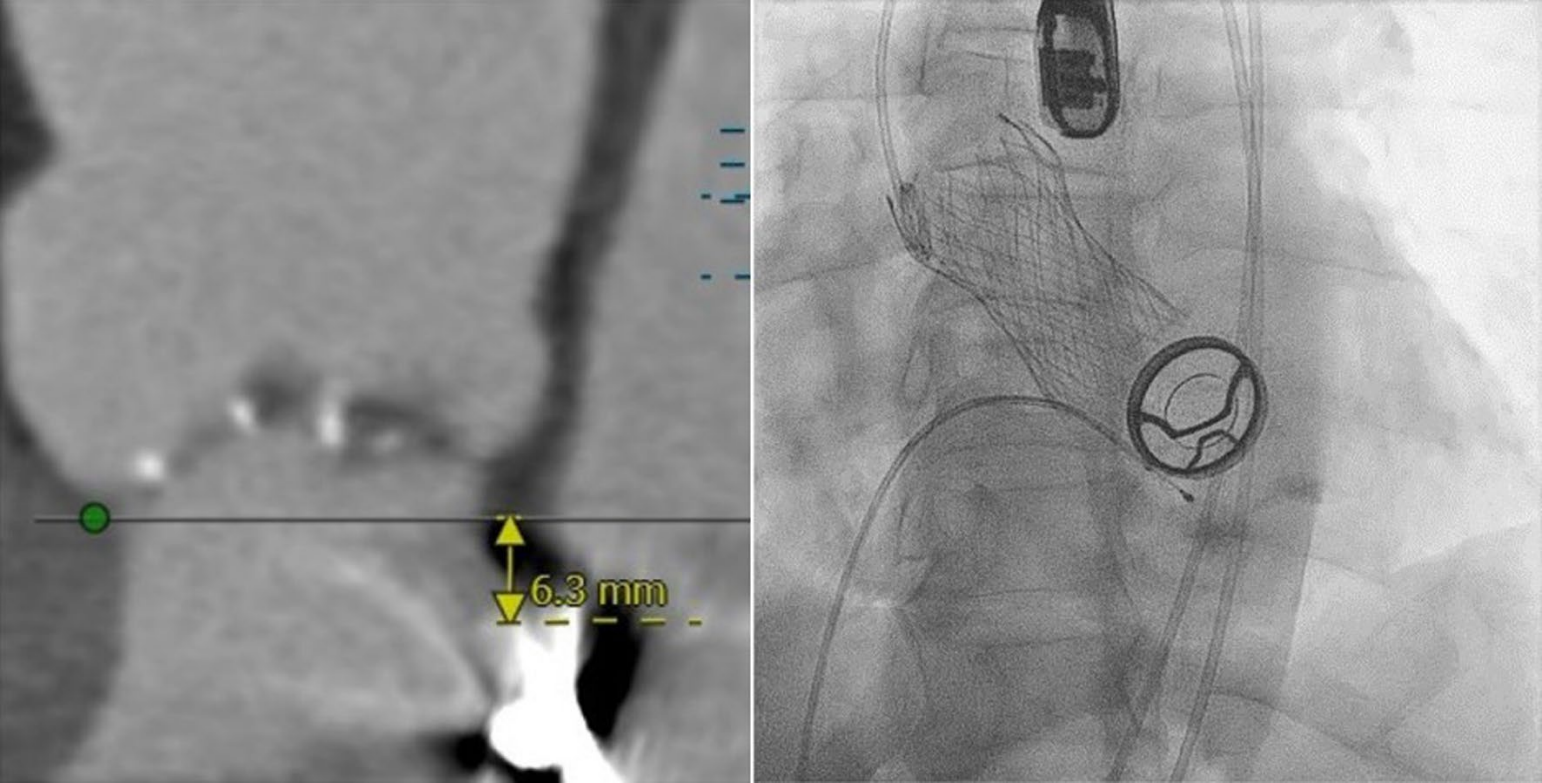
(case 1): On the left, pre-procedure Cardiac CT scan showing the prosthetic mitral valve—aortic annular distance. On the right, post-procedure fluoroscopic image showing deployed self-expandable THV in the aortic position and a mechanical PHV in the mitral position
